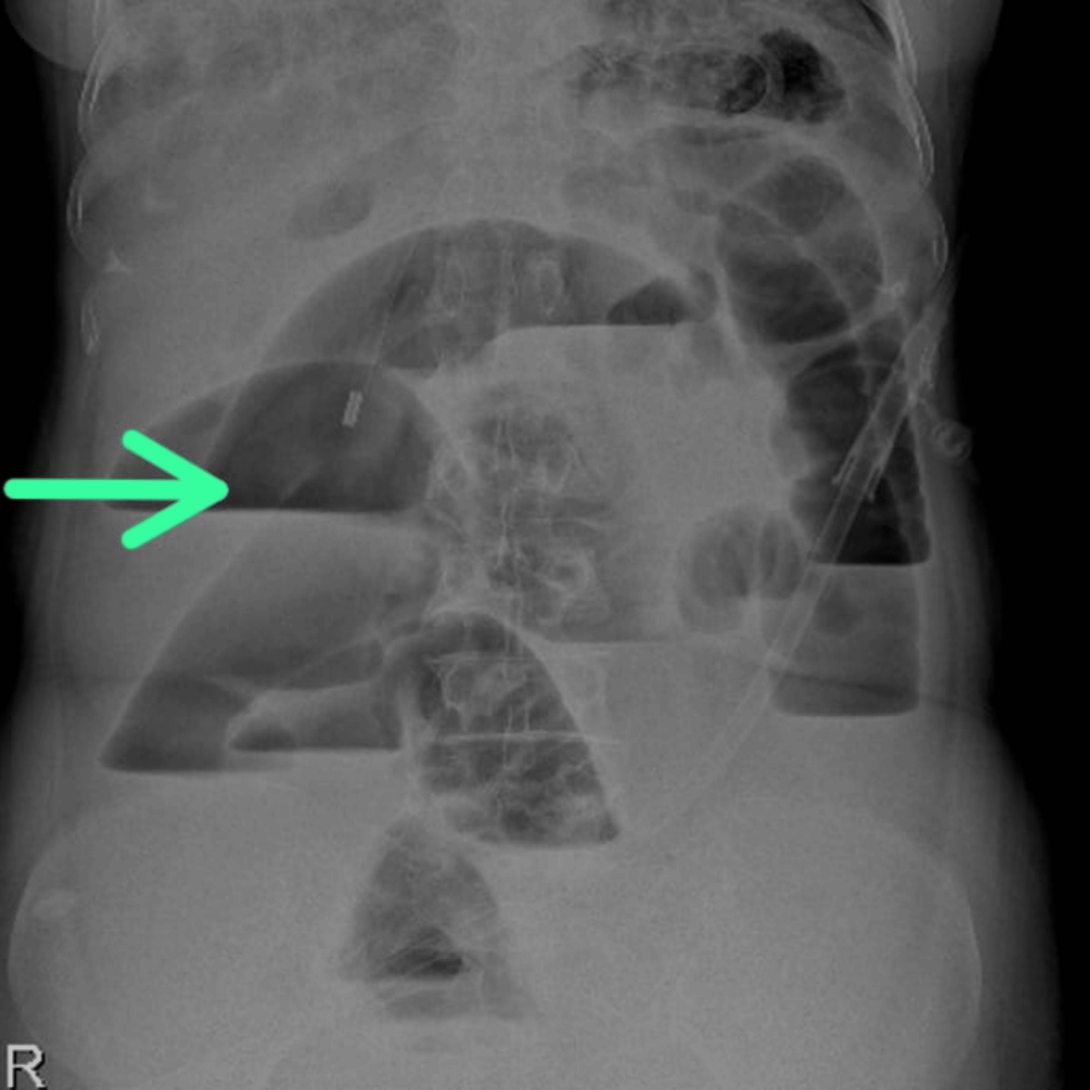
Radiograph of the Erect Abdomen Showing Multiple Air Fluid Levels
